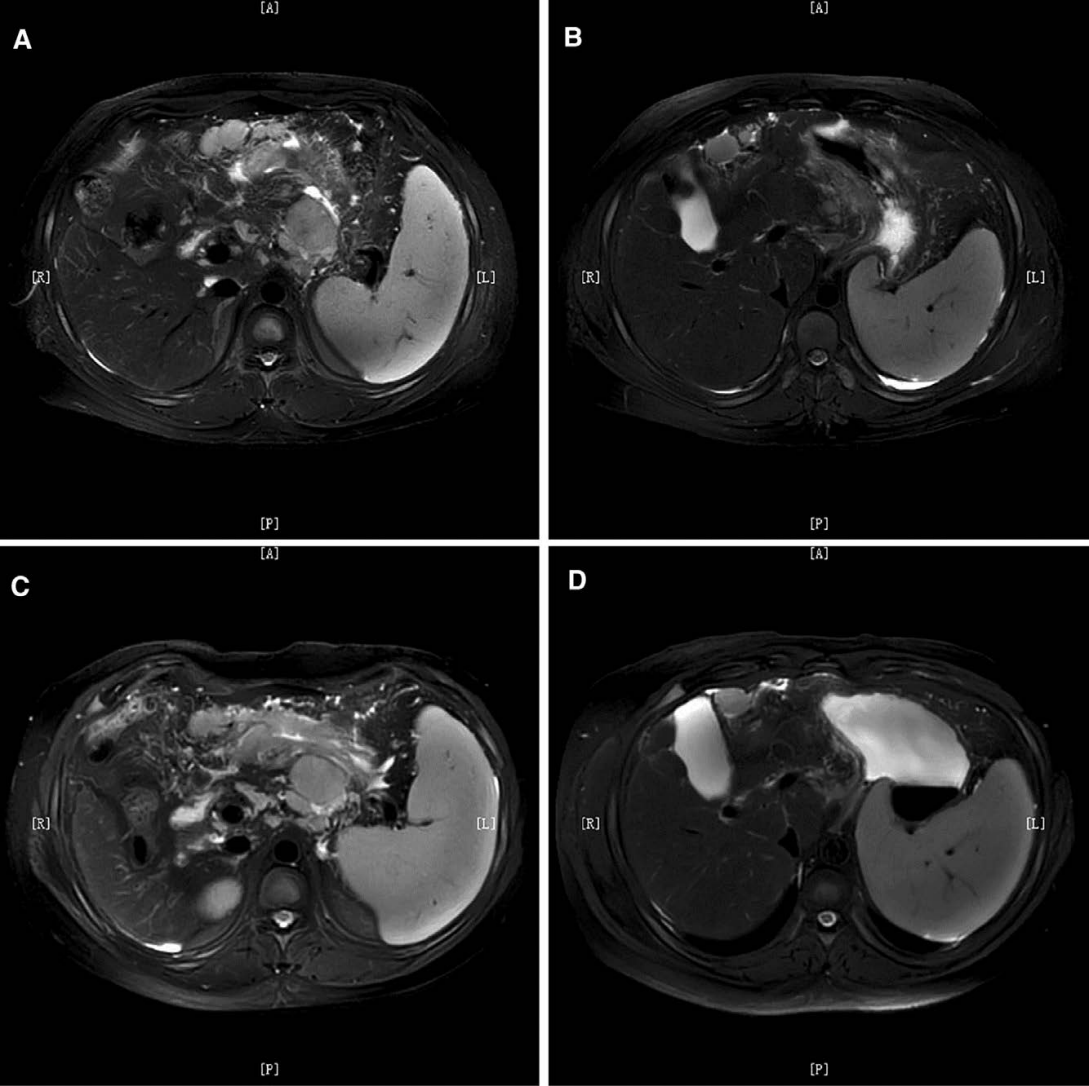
Before treatment, MRI showed uneven thickening of the stomach wall and multiple enlarged lymph nodes in the hepato-gastric space and retroperitoneum, the largest being approximately 4.3 × 4.0 cm (A, B). After 3 cycles of mFOLFOX6 chemotherapy, MRI showed decreased thickness of the gastric wall lesions and some reduction of lymph nodes, the largest being 4.0 × 3.7 cm (C, D).
